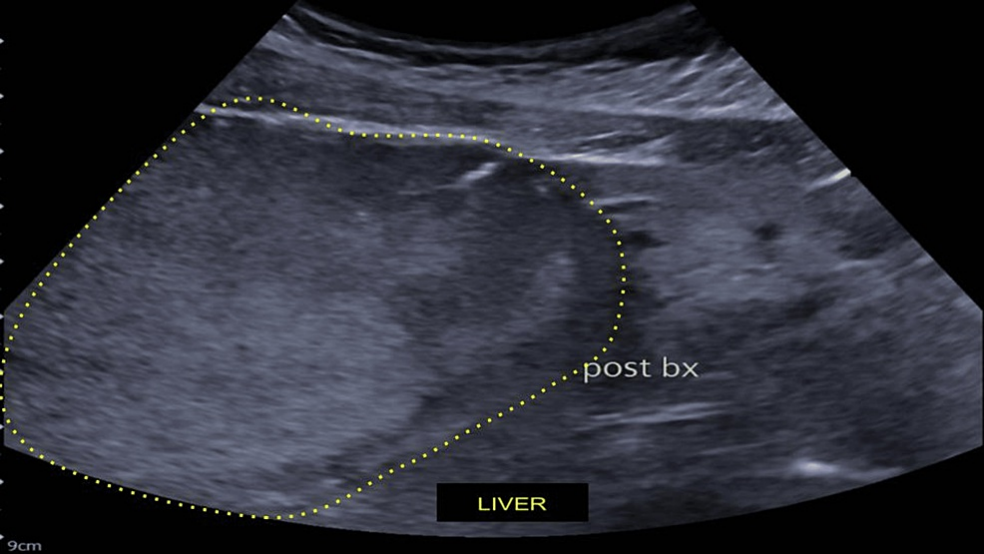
Liver appearance post-ultrasound-guided biopsy. No hematoma detected after biopsy.
